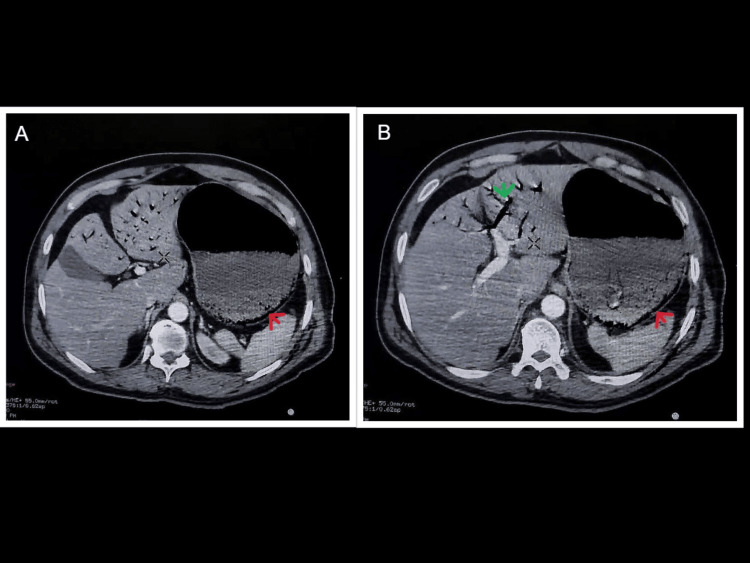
Axial section of CECT whole abdomen A. Gastric pneumatosis marked with a red arrow. B. Portal venous gas marked with a green arrow and gastric pneumatosis with a red arrow. CECT, contrast-enhanced computed tomography.
